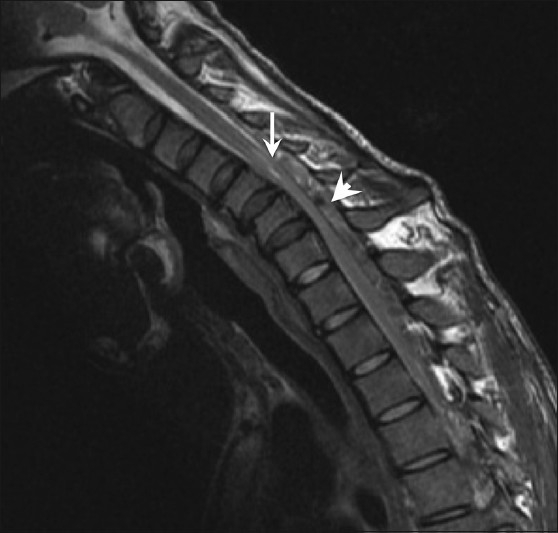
T2W sagittal magnetic resonance image in flexion shows anterior displacement of the dorsal dura (arrow) compressing the thecal sac, with a prominent dorsal epidural compartment (arrowhead)
